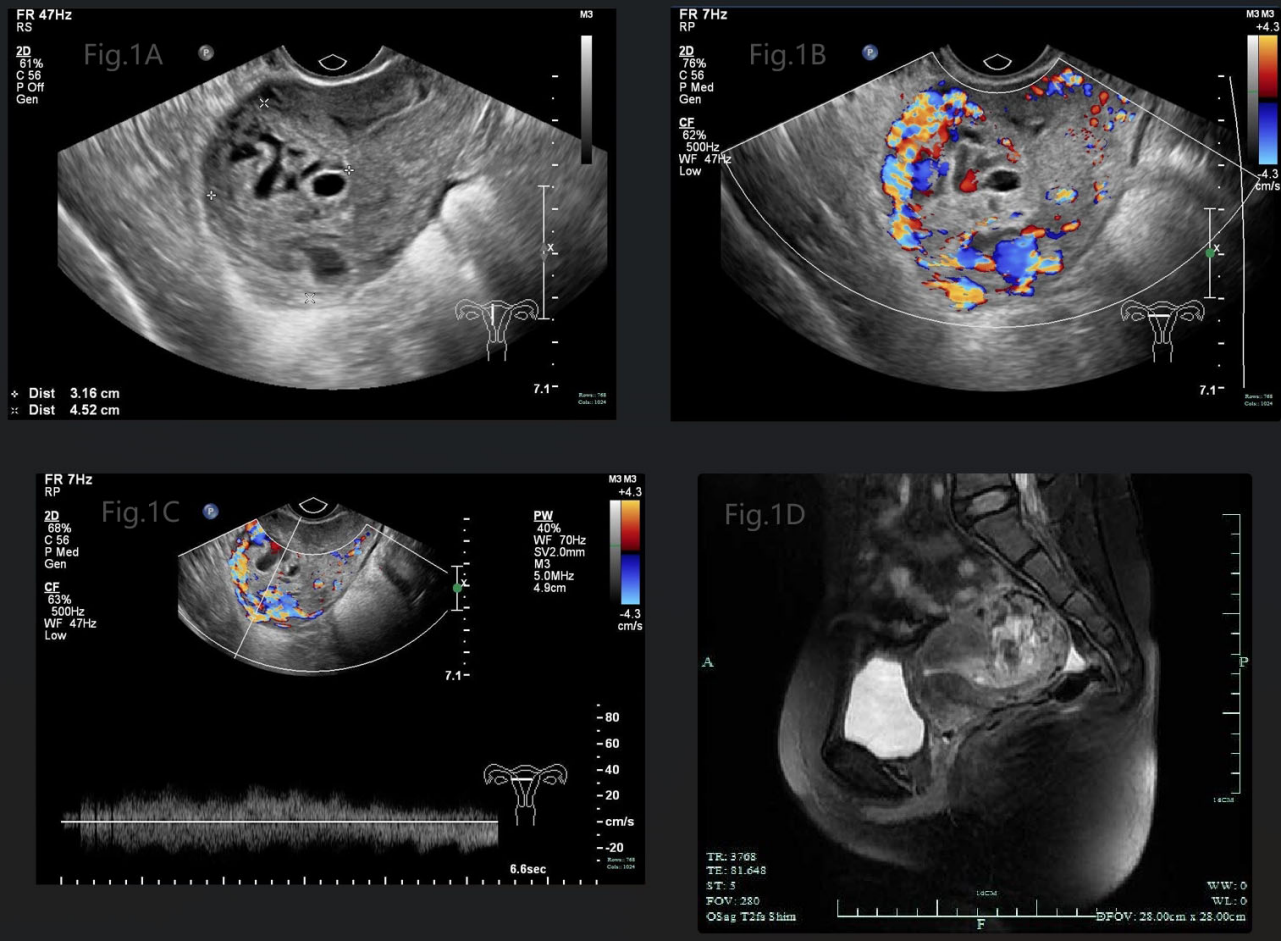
(A) Gray-scale pelvic sonography displayed a mixed echogenic mass in the uterine cavity, partially invading the myometrium, with honeycomb like internal echoes. (B) Color Doppler showed that the mass was filled with abundant blood flow signals. (C) Spectrum of arteriovenous fistula was measured in pulsed-wave Doppler. (D) Pelvic MRI scan depicted heterogeneous mass in uterine cavity with hemorrhage, invading to the myometrium.
